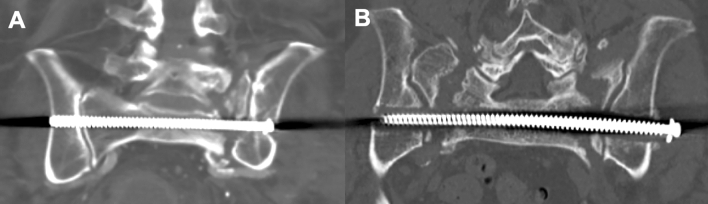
Pseudarthrosis after 6 months in CT-scan without loosening (A) and with minor loosening of a TSTI screw (B)
